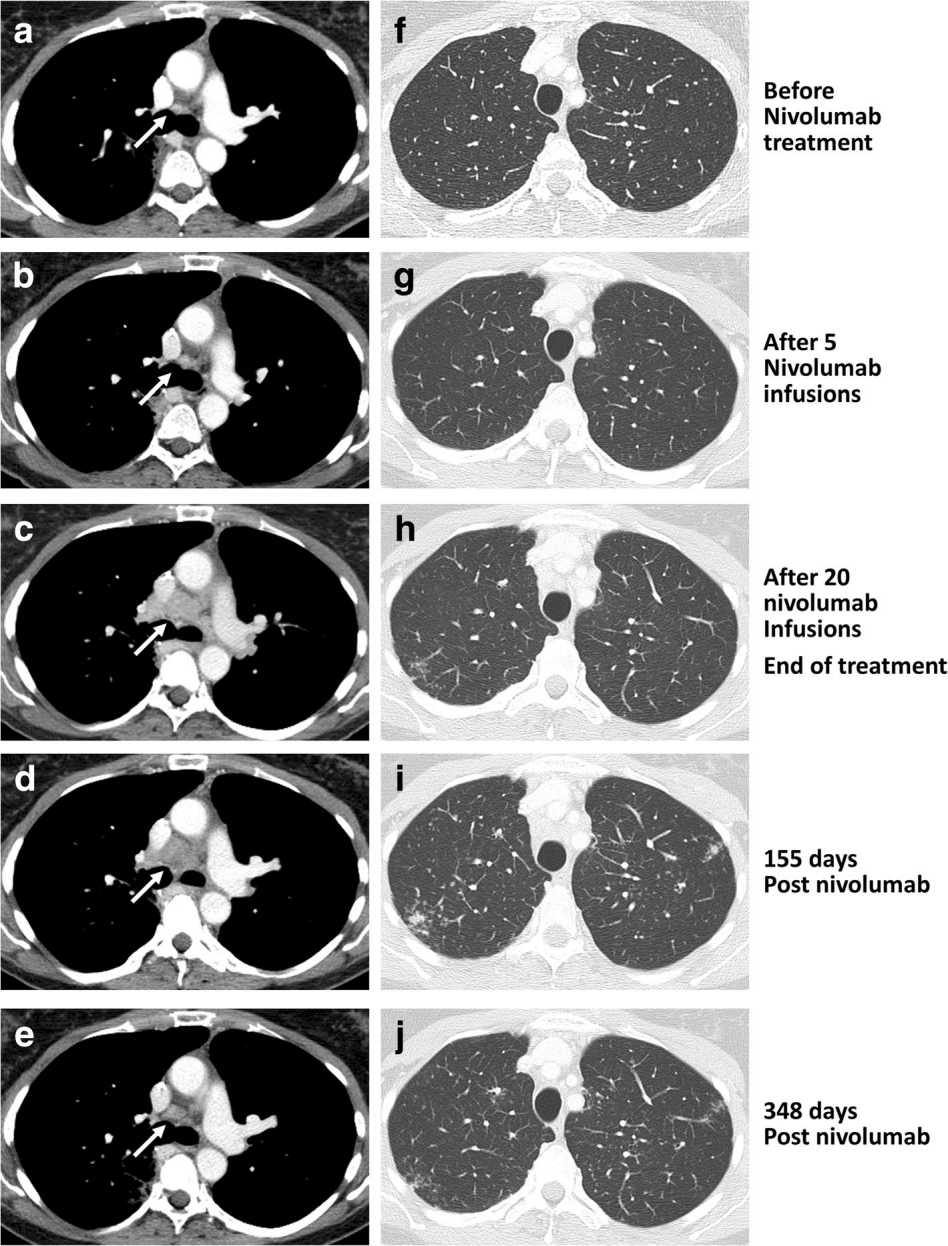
Measure of the size of mediastinal lymph nodes and interstitial syndrome. Plain chest computed tomography of the index case was carried out at different time before and after treatment discontinuation. The mediastinal lymph nodes (white arrows) measurement was achieved with a mediastinal-window setting (left panels, a-e) whereas interstitial syndrome was evaluated with a lung-window setting (right panels, f-j)
